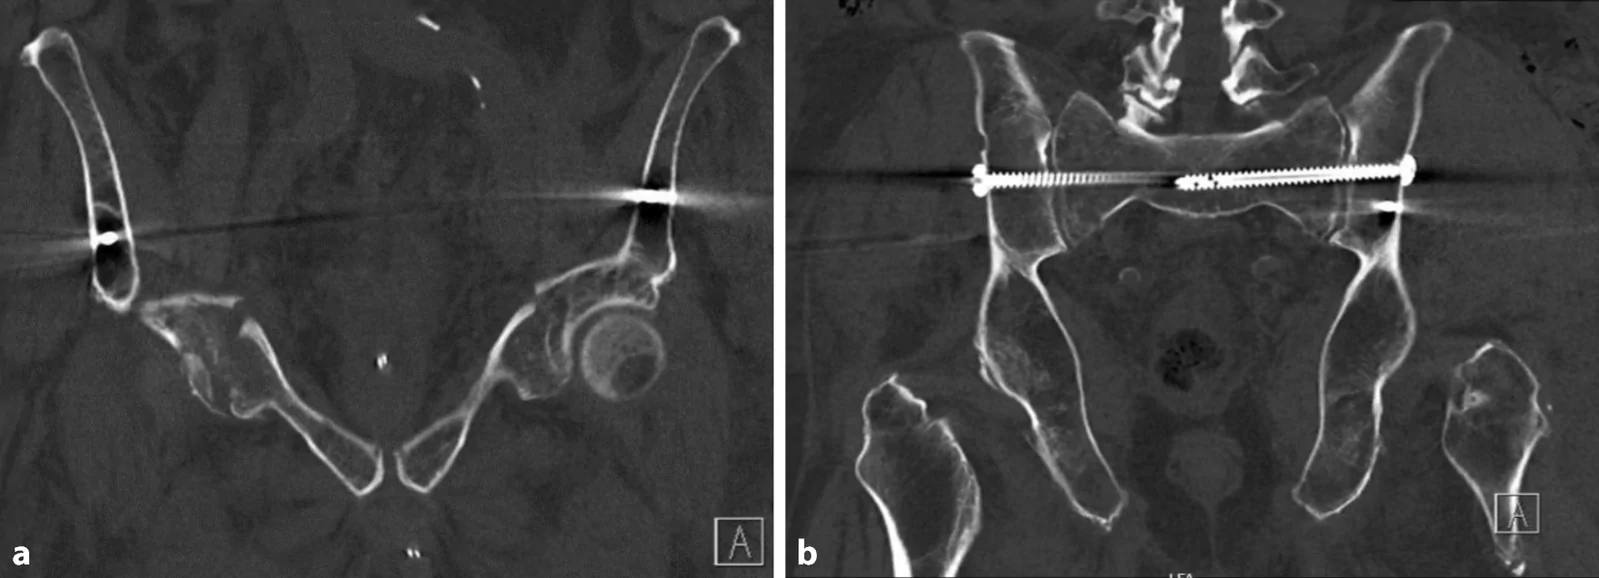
Postoperatives CT mit einliegendem supraacetabulärem Fixateur externe und beidseitig eingebrachten Iliosakralschrauben (a und b koronar)
